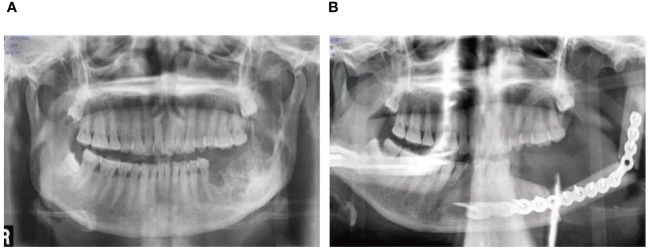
Repair and reconstruction of peroneal musculocutaneous flap. (A) Panoramic radiograph before treatment; (B) Panoramic radiograph after treatment.
